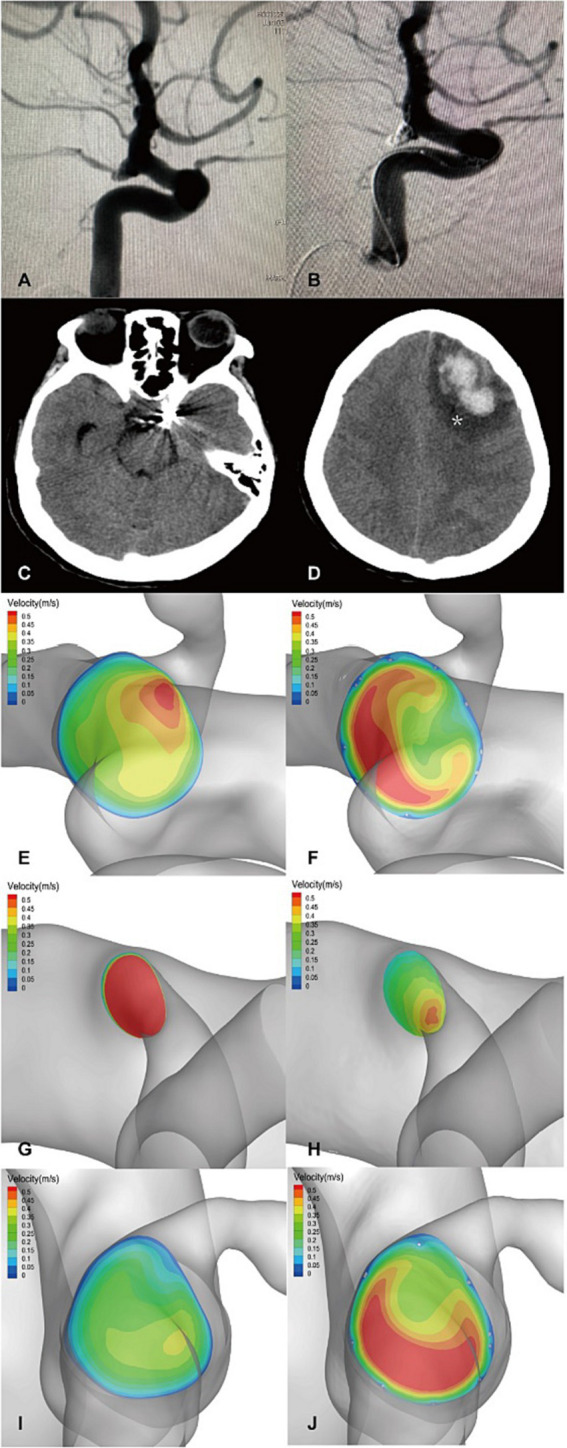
A posterior communicating segment aneurysm of left ICA in DICH of type 1 was treated with EP2 stent assisted coiling. (A) Preprocedural angiography of the aneurysm and (B) postprocedural immediate angiography revealed Raymond grade 2. (C) The coiled aneurysm was showed by CT scan at 24 h later after the treatment. (D) CT scan obtained 4 days post-procedure, revealing ipsilateral frontal lobe DICH with surrounding infarcted area (asterisk). A significant increase in velocity was observed on the terminal section of the ICA (F) compared to the preoperative results (E) by computer hemodynamic detection (Increase rate was 44.9%). The velocity of DICH related artery (ACA) decreased significantly after embolization with increase rate of-35.9% (G,H), while the flow velocity of DICH unrelated artery (MCA) increased with increase rate of 35.7% (I,J). The imbalance index of this patient was 71.6%.
